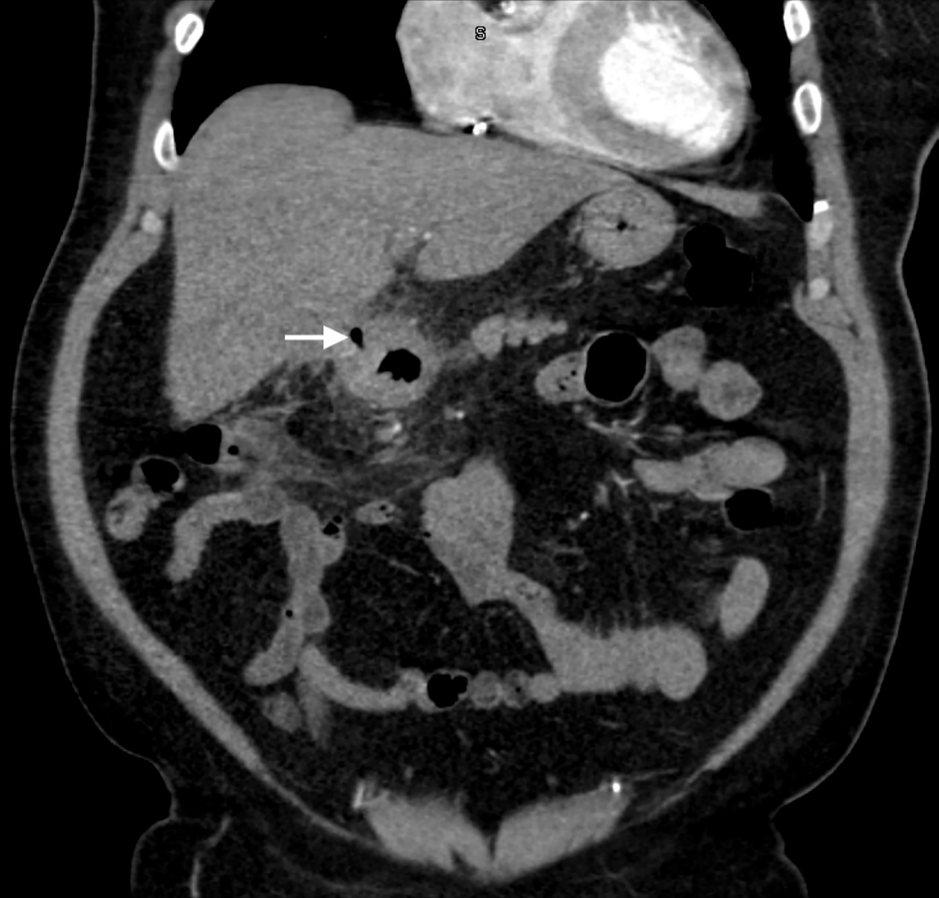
Computed tomography angiography (coronal view) demonstrating a wide, soft tissue tract between the gallbladder and proximal duodenum, containing a small amount of air (white arrow).
